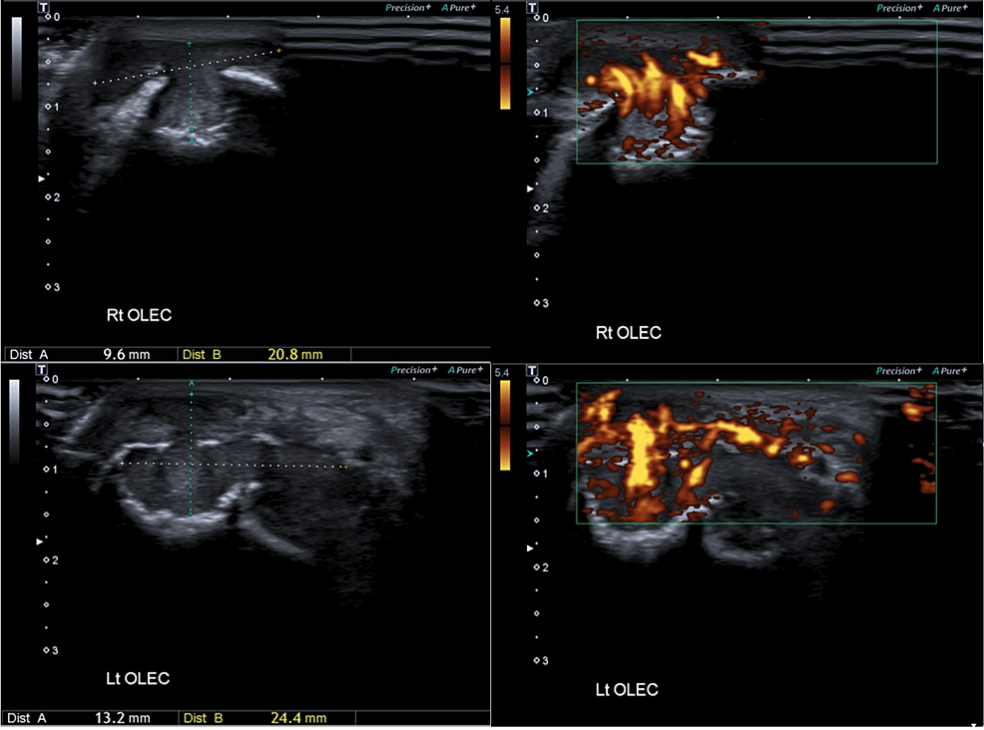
Ultrasound Elbow: There is evidence of abnormal soft tissue thickening in the olecranon regions bilaterally, worse on the left. The soft tissue swelling measures 13 x 25 x 33 mm on the left and 10 x 21 x 19 mm on the right. Bilateral erosions of the olecranon are noted. There is severe increased vascularity in the described soft tissue thickening bilaterally.
